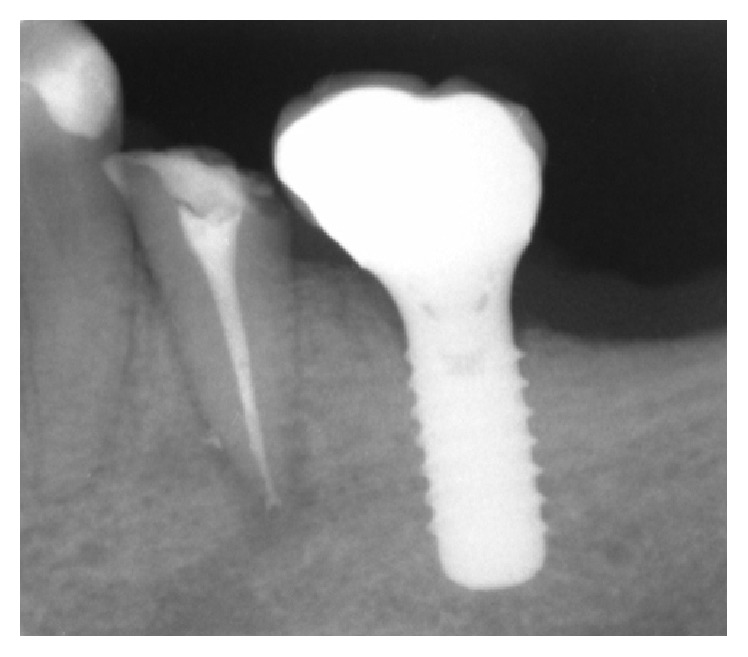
Radiographic control after endodontic treatment.
